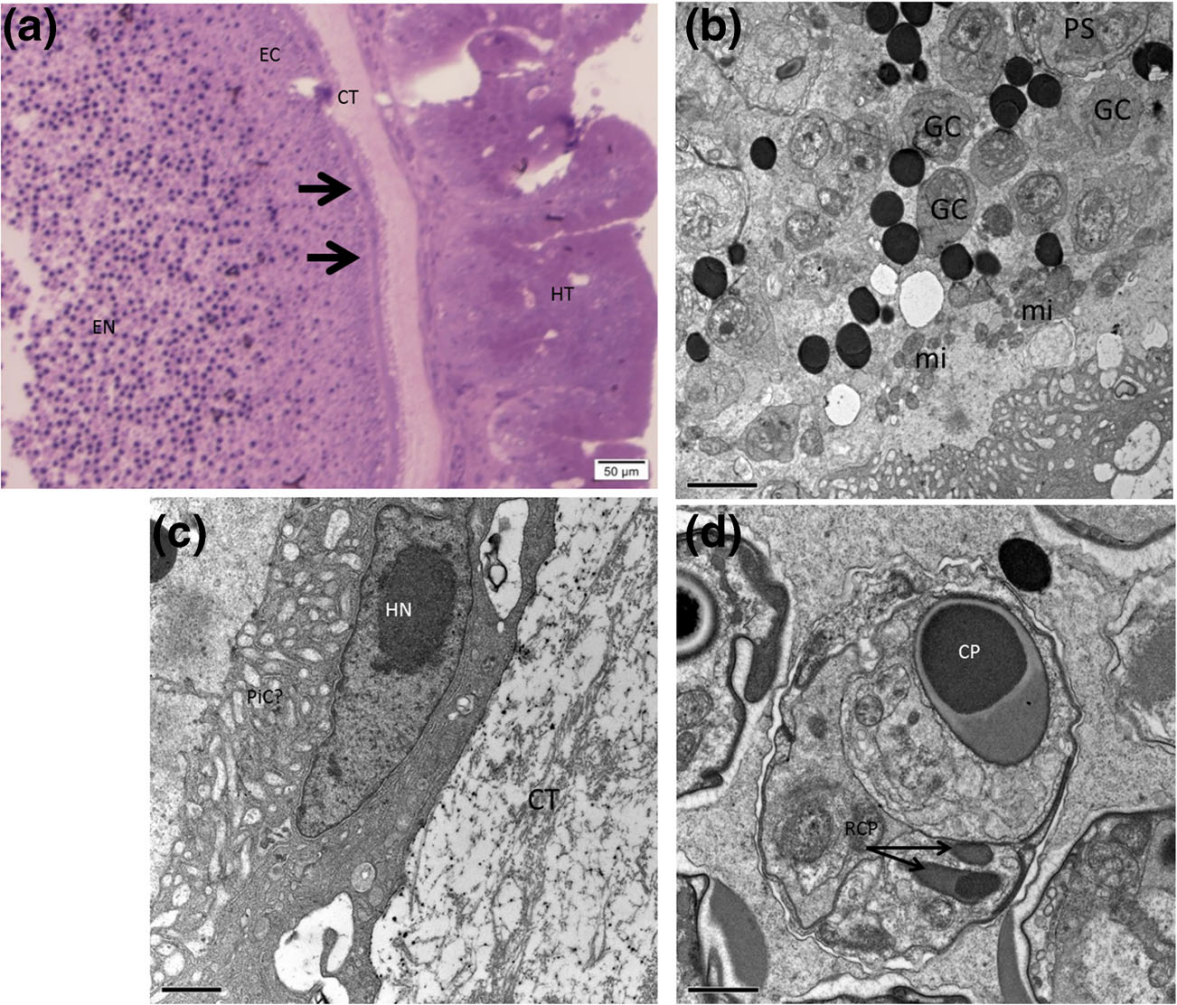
a Semi-thin section through a cyst showing the division of the cyst complex into several layers, the endoplasmic region (EN), ectoplasmic region (EC), peripheral membrane (arrows), connective tissue (CT), and host tissue (HT). b Ultrathin section of the plasmodia showing a close-up of the plasmodia and host interface with details of the peripheral membrane which is located between the ectoplasmic region and the connective tissue wall; note the host cell (*asterisk*) with the nucleus (HN) trapped inside the membrane and the several arms or web- like structure which possibly could be pinocytotic channels (PiC). c Details of the ectoplasmic region with several single nucleus *GC* generative cells, *PS* Pansporoblast, *mi* mitochondria, lipid droplets, and young spores. d Young spore with a developing polar capsule or *CP* capsular perimordium of the large polar capsule. Furthermore, note the two structures (arrows), which appear to be perimordium of the *RCP* rudimentary polar capsules. Scale bar for b, c, d = 1.0, 2.0, and 1.0 μm, respectively
